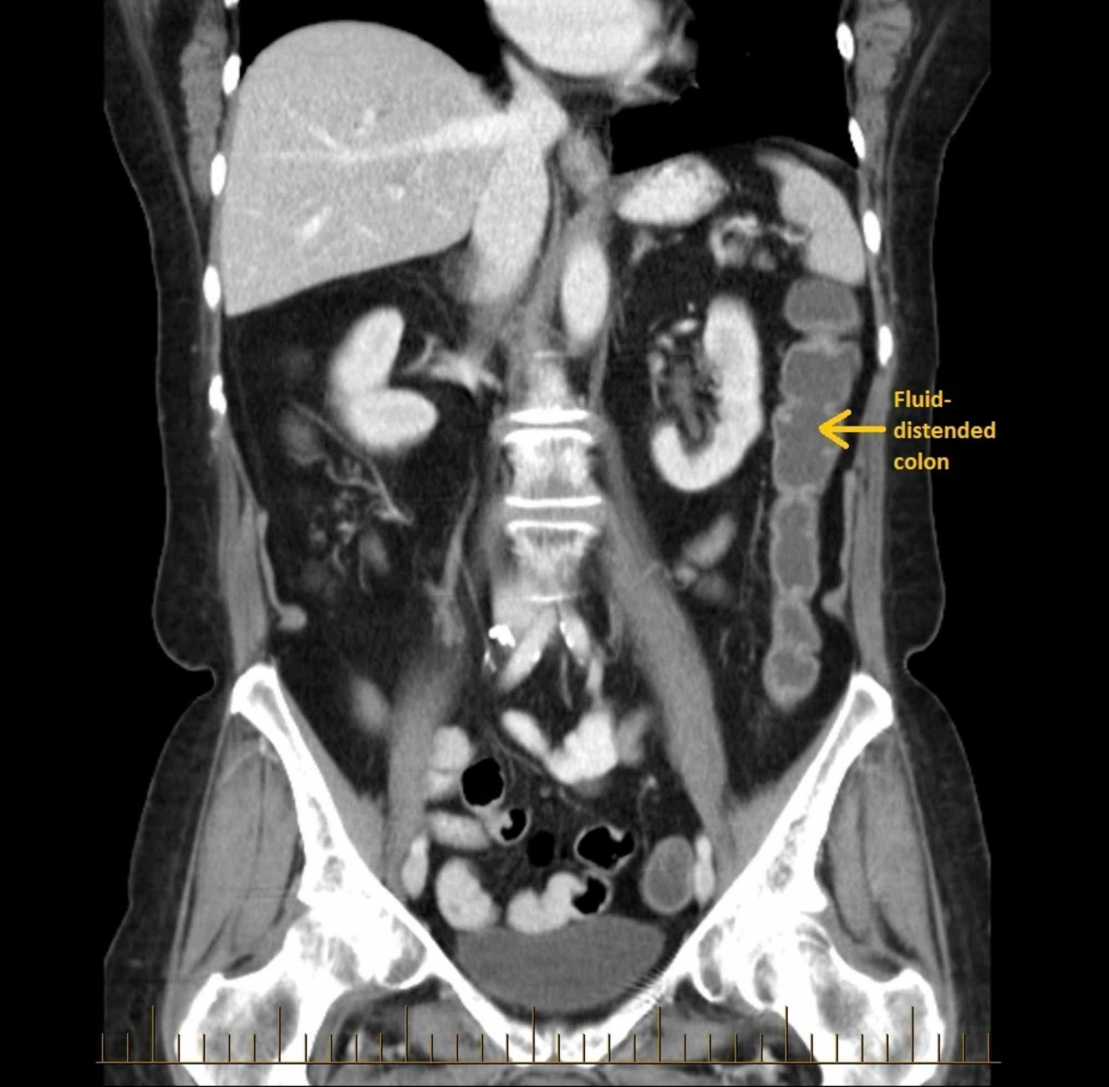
Abdominal CT scan whilst symptomatic on olmesartan showed generalized borderline fluid-filled distension of the colon without thickening or concerning focal lesion of the bowel and no adjacent fat stranding or perforation.
